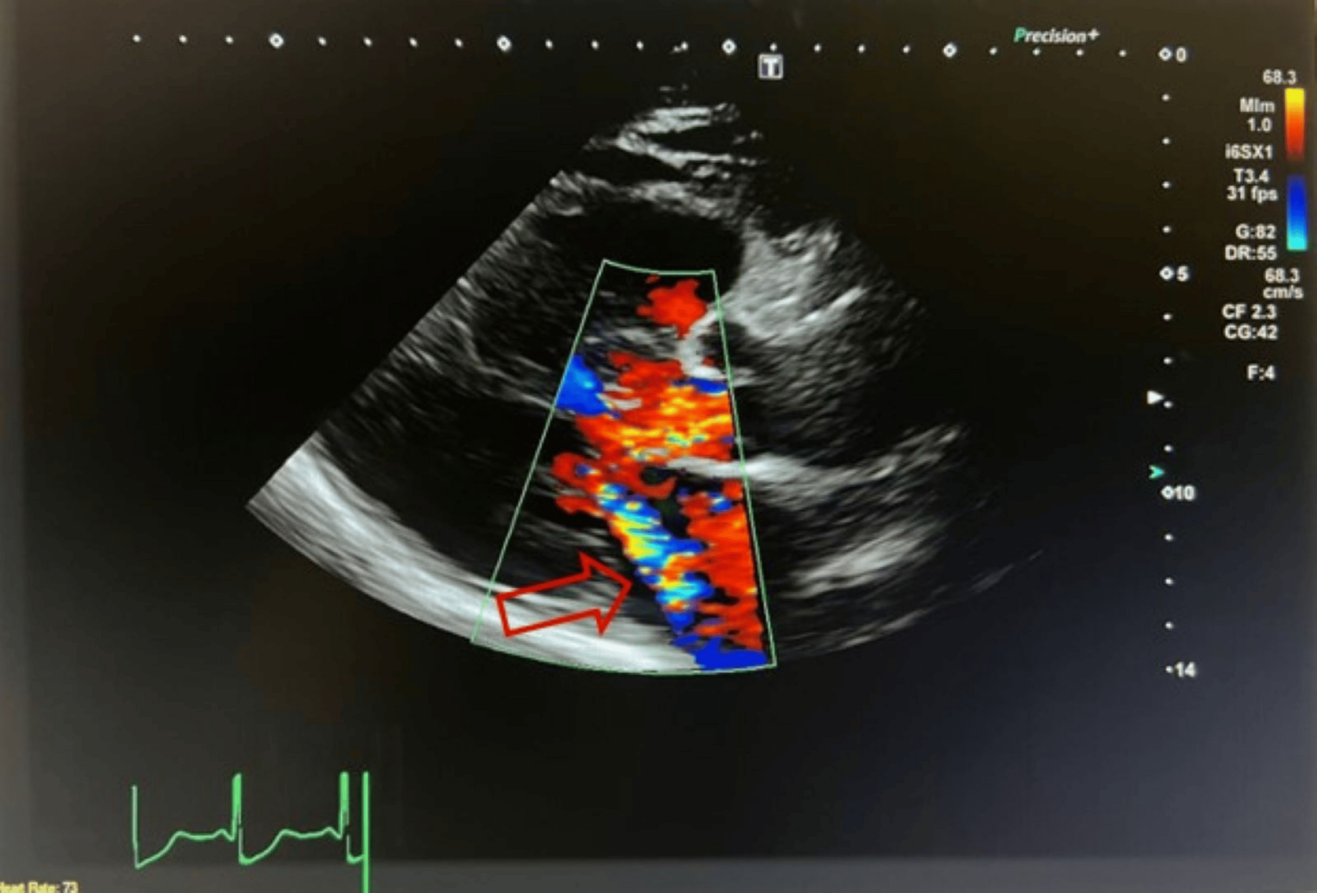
Transthoracic echocardiogram in a parasternal view showed posteriorly directed mitral regurgitation, typically seen in severe left ventricular hypertrophy with systolic anterior motion of the anterior leaflet of the mitral valve (red arrow).
